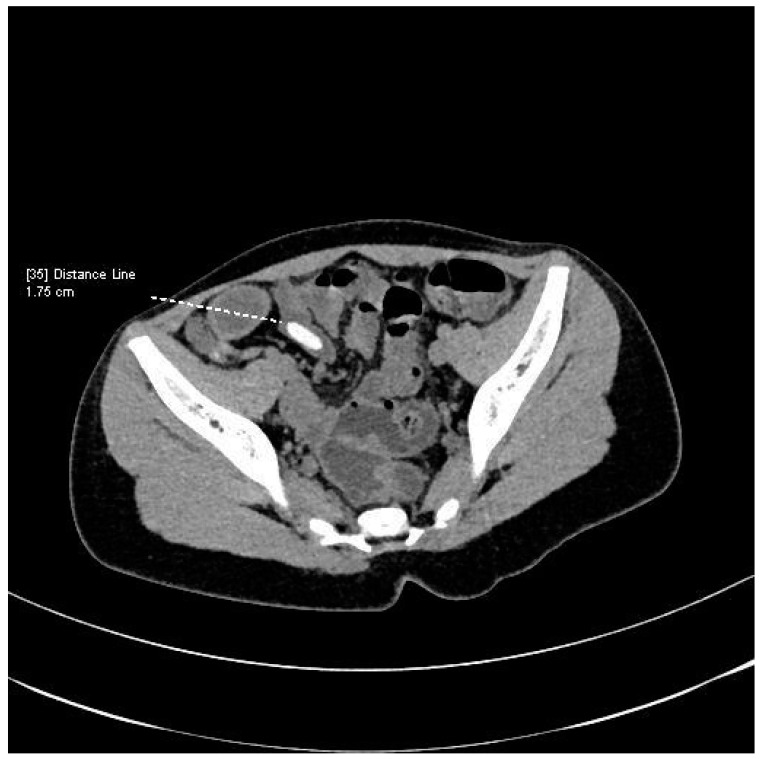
CT scan of the abdomen and pelvis.
